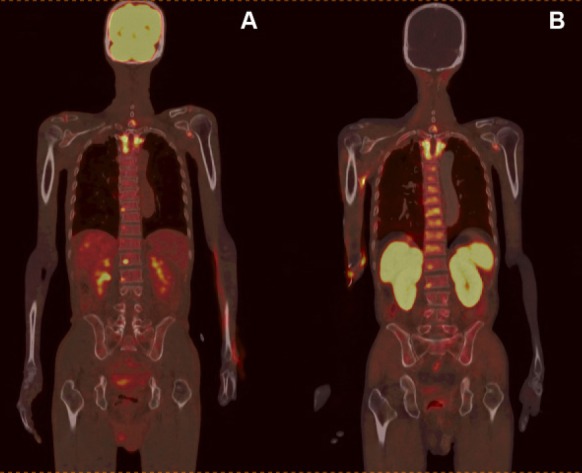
Bone metastases in the upper thoracic spine detected by both A) ^18^F-FDG and B) ^11^C-choline PET/CT
